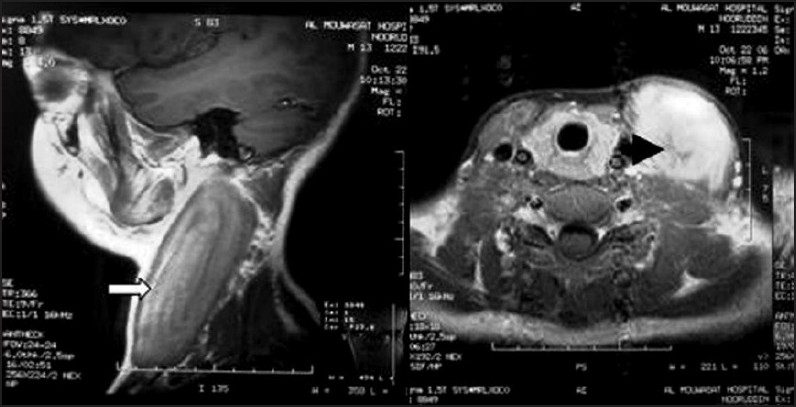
MRI of neck showing diffuse enlargement of left SCM (isointense on T1 (open arrow) and mildly hyperintense on T2 and enhancing after IV contrast (block arrow).
